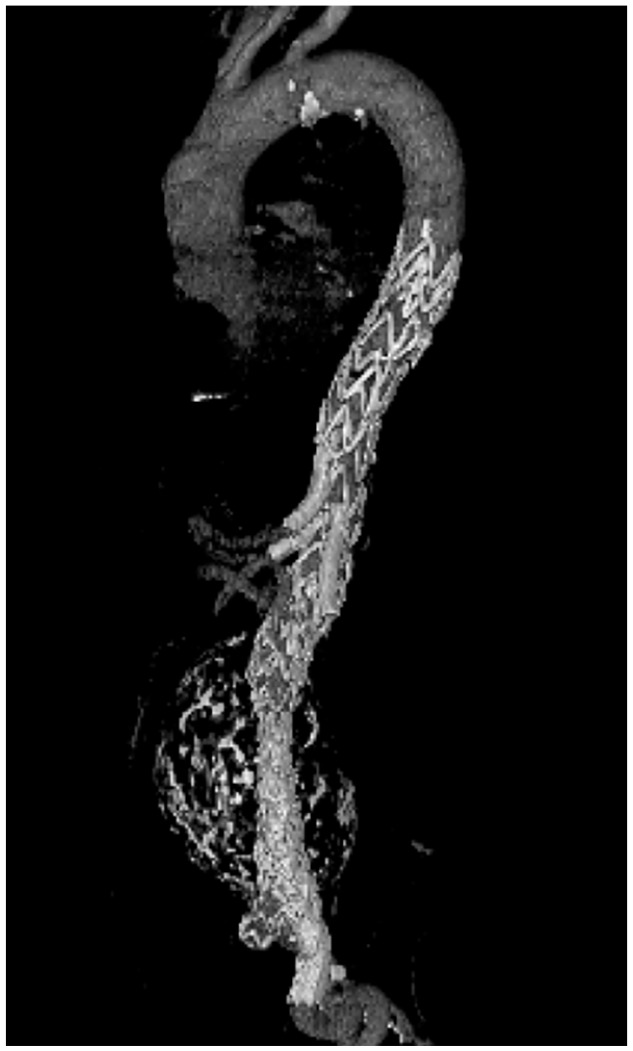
Postoperative volume rendering of a computed tomography angiography of a successfully implanted E-nside stent graft.
